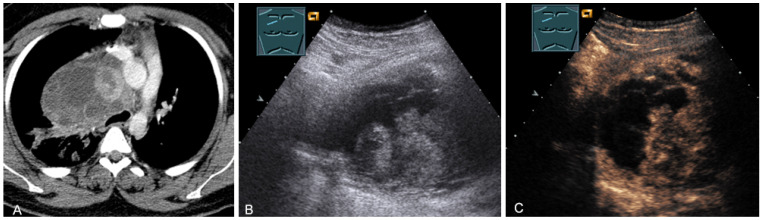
A patient, with a history of germ cell tumor, with tumor formation in the anterior mediastinum on CT (provided by Prof. Dr. Andreas H. Mahnken, Marburg, Germany) (A), B-mode US (B), and CEUS (C), confirmed by biopsy as mediastinal metastasis of malignant teratoma.
